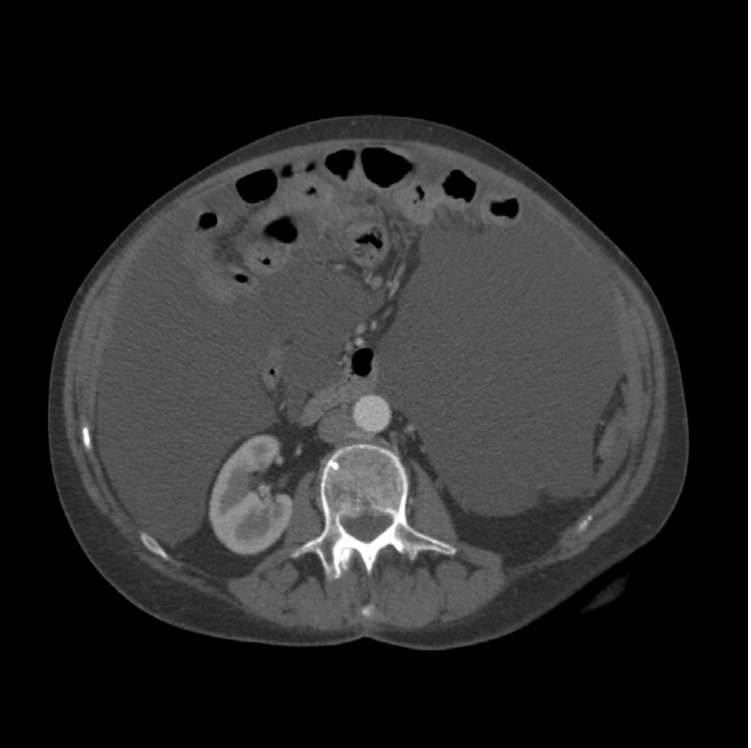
CT scan of the abdomen showing ascites.
